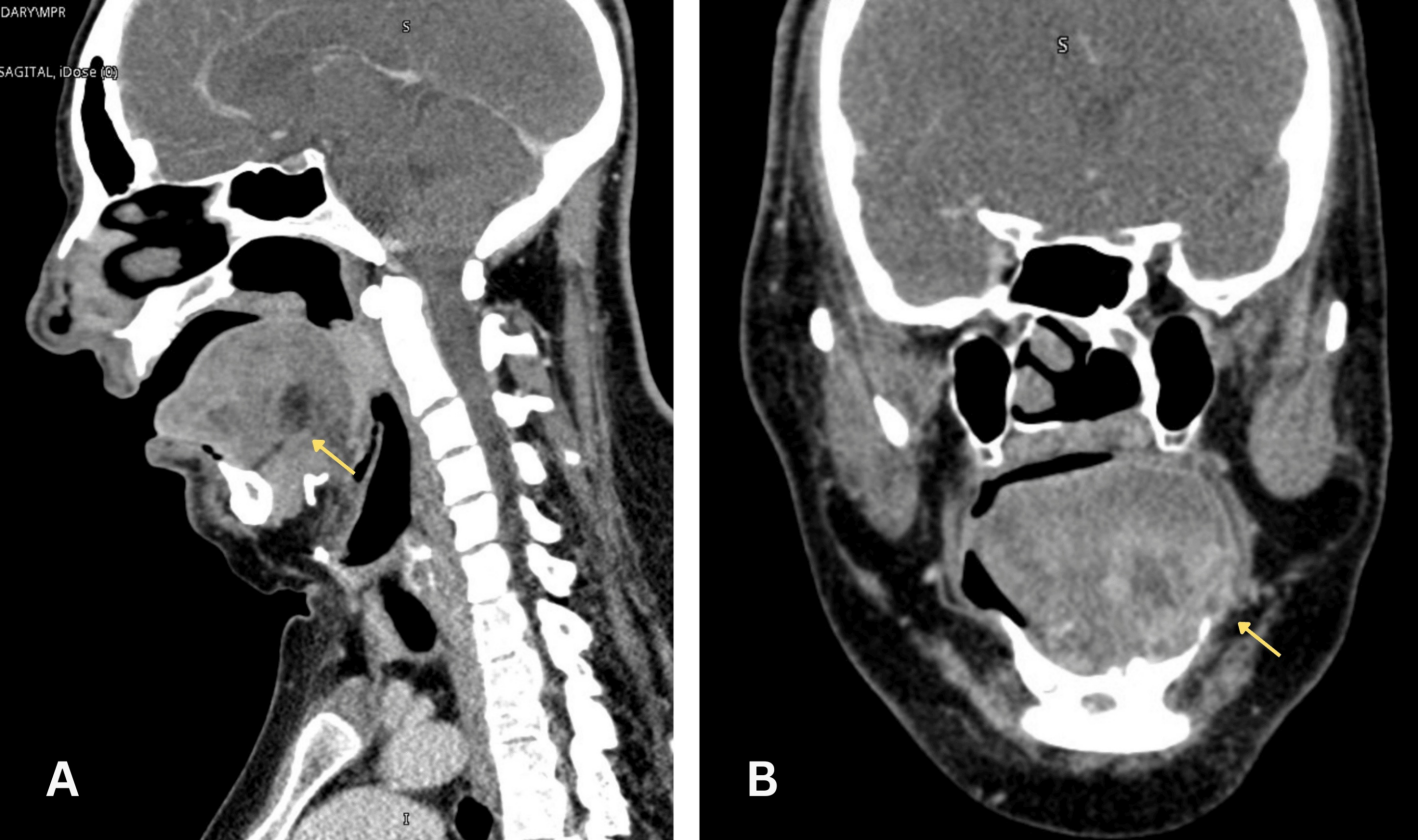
Contrast CT neck scan. Collection in the left hemitongue involving the intrinsic muscles. (A) Sagittal and (B) coronal window (yellow arrow) show collection with periferial enhancement.
